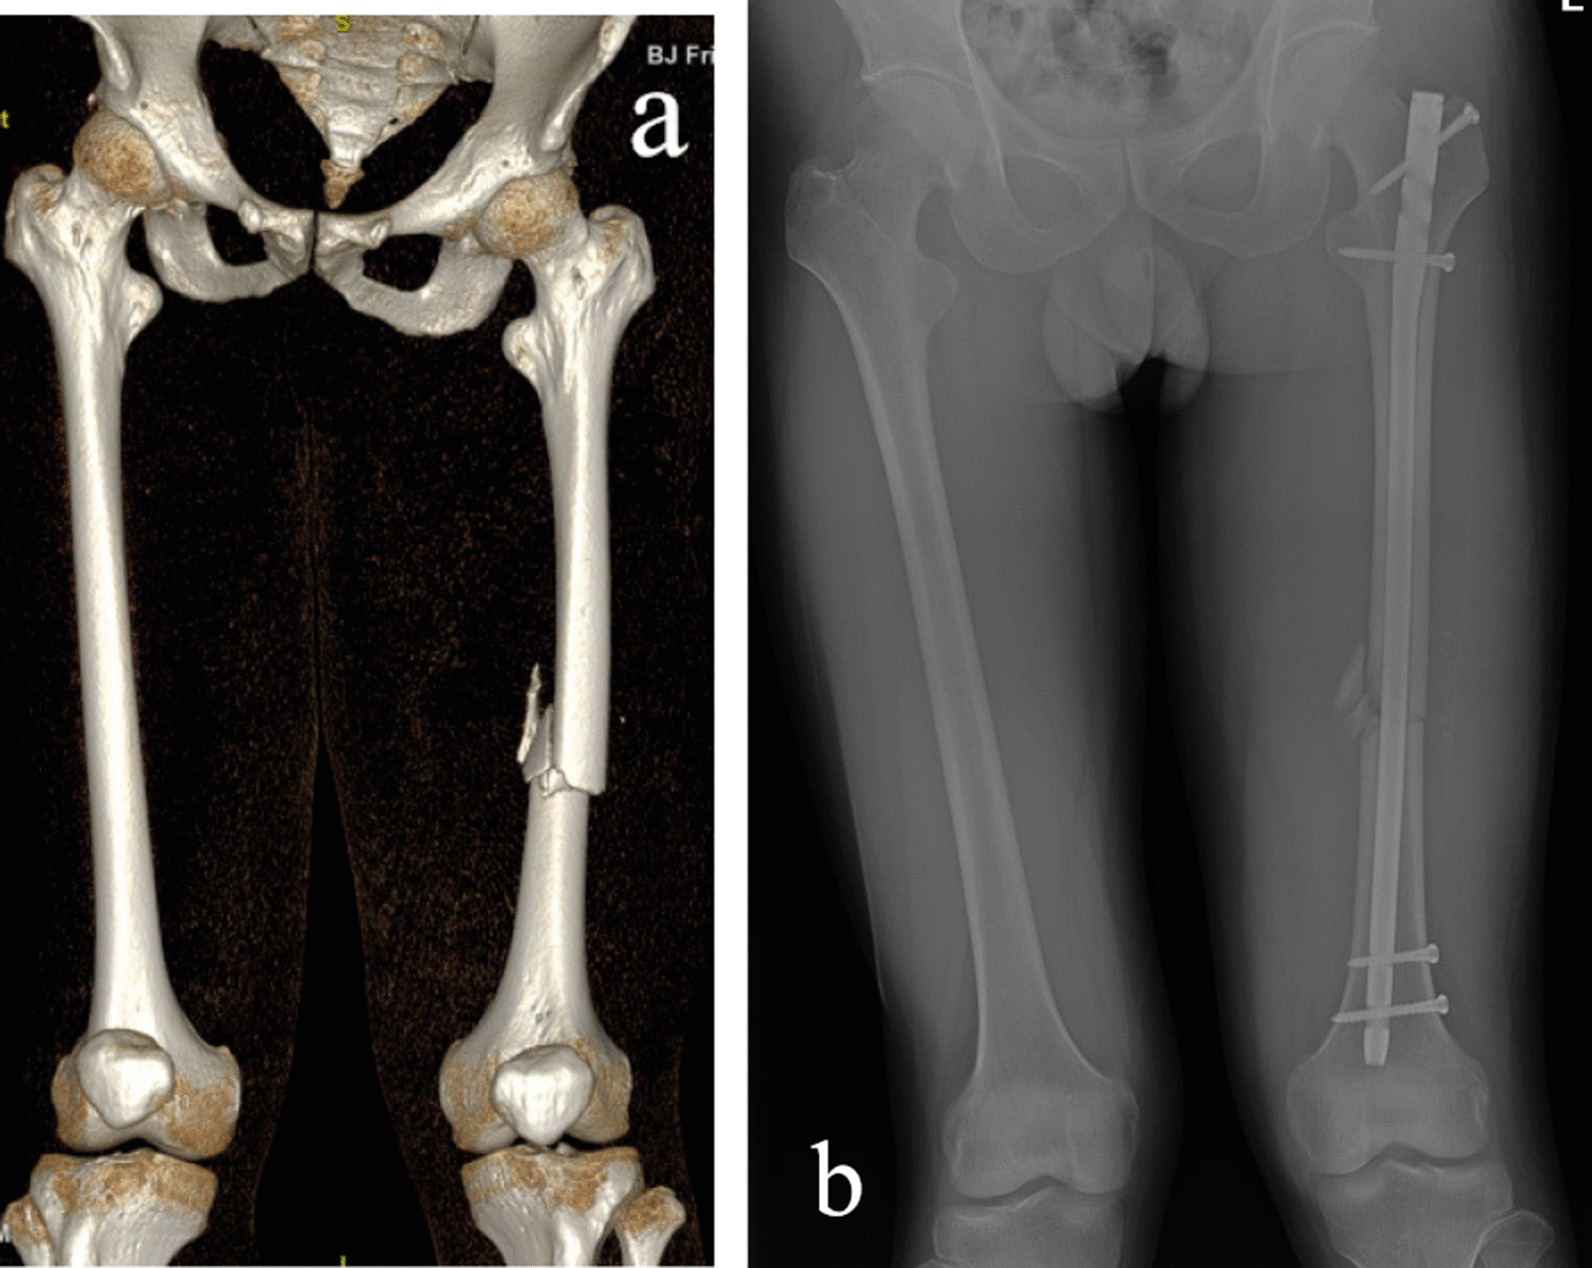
Preoperative three-dimensional CT image of a patient with femoral shaft fracture (a). X-ray image of this patient undertaken IMN with the laser guiding navigation device on the first day of postoperative (b)
